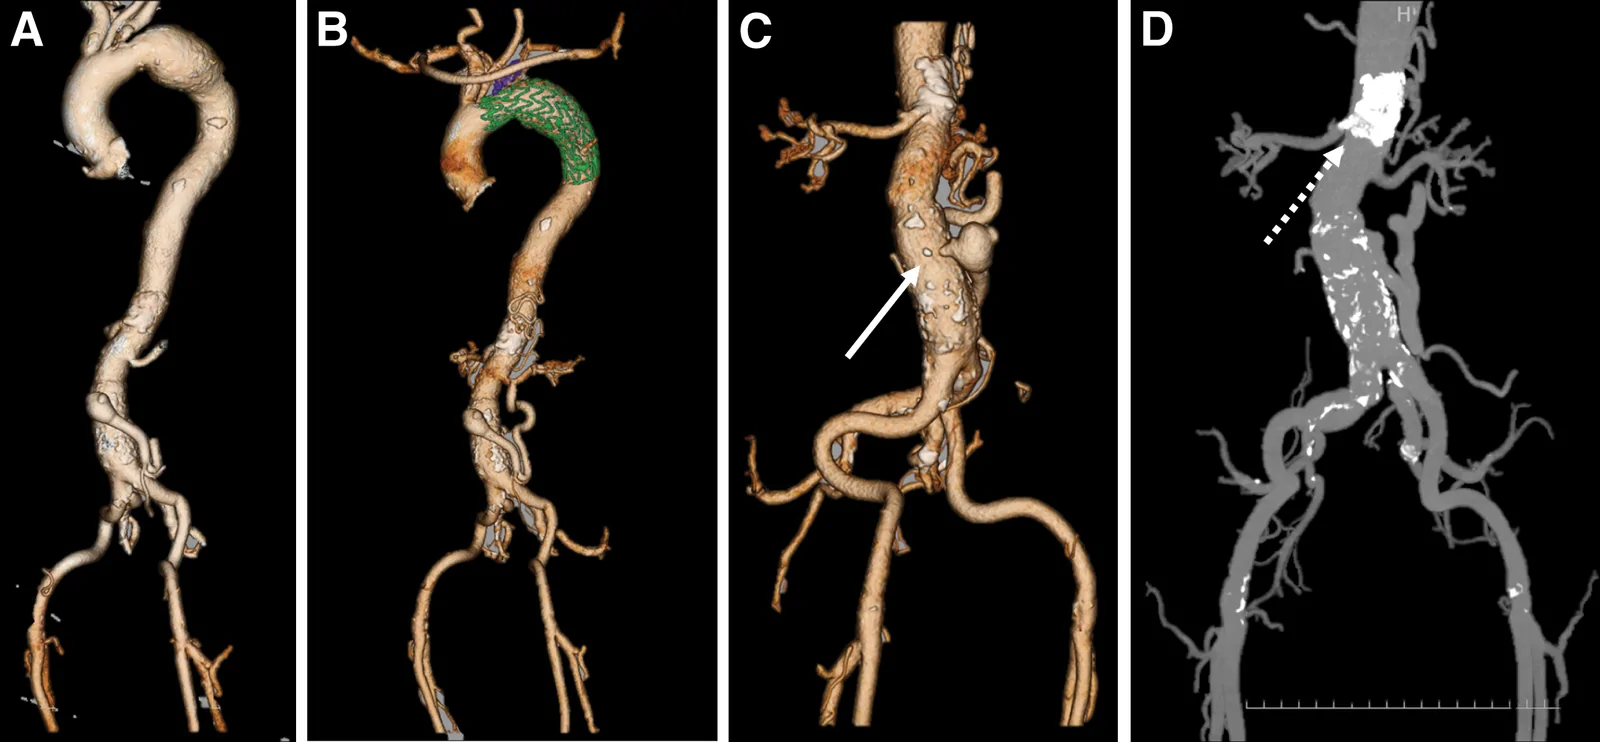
(A) 3D CTA revealed a TAA measuring 5.6 cm, an IMA aneurysm measuring 3.0 cm, and CTO of the CA and SMA. (B) Debranching TEVAR was performed as the first-stage procedure for the TAA. (C) 3D CTA viewed from a slight right anterior oblique angle demonstrates the spatial relationship between the saccular IMA aneurysm and the abdominal aorta and the absence of an adequate clamping zone at the IMA orifice (arrow). (D) Extensive aortic calcification obstructing the orifices of the CA and SMA (dotted arrow). CA, celiac artery; CTO, chronic total occlusion; IMA, inferior mesenteric artery; SMA, superior mesenteric artery; TAA, thoracic aortic aneurysm; TEVAR, thoracic endovascular aortic repair
